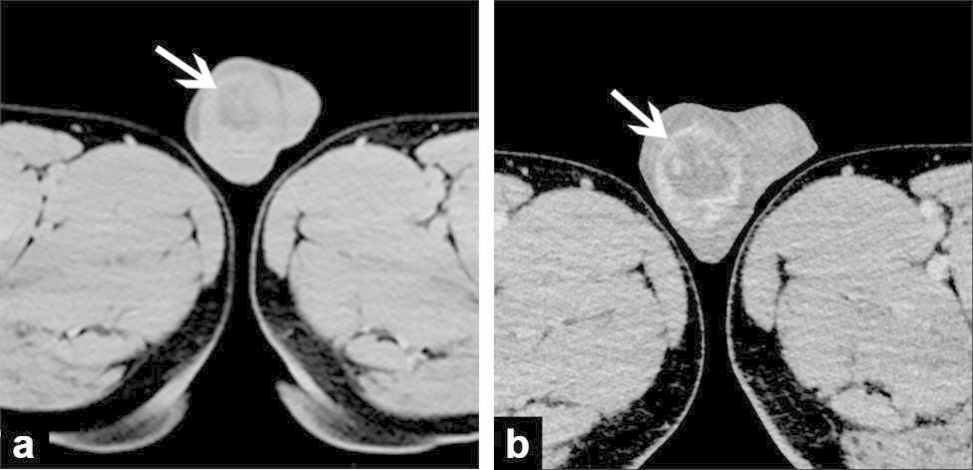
Computed tomography images. (a) plain and (b) enhanced CT showing a right testicular mass with heterogeneous density and enhancement
